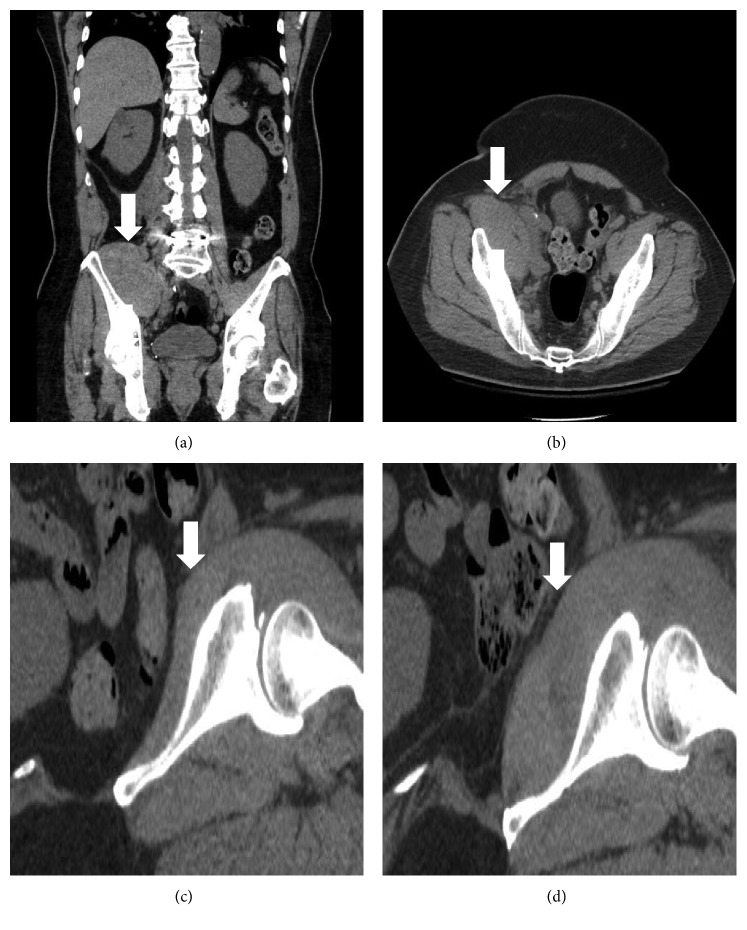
Computed tomography scan of the abdomen and pelvis. Coronal (a) and axial (b) planes show asymmetrically enlarged right iliacus muscle (white arrows), consistent with hematoma. Sagittal plane of the hips: left unaffected hip (c) in comparison to the right hip (d), which showed right psoas muscle bleed after treatment of recombinant human factor VIIa and Factor Eight Inhibitor Bypassing Activity (FEIBA).
